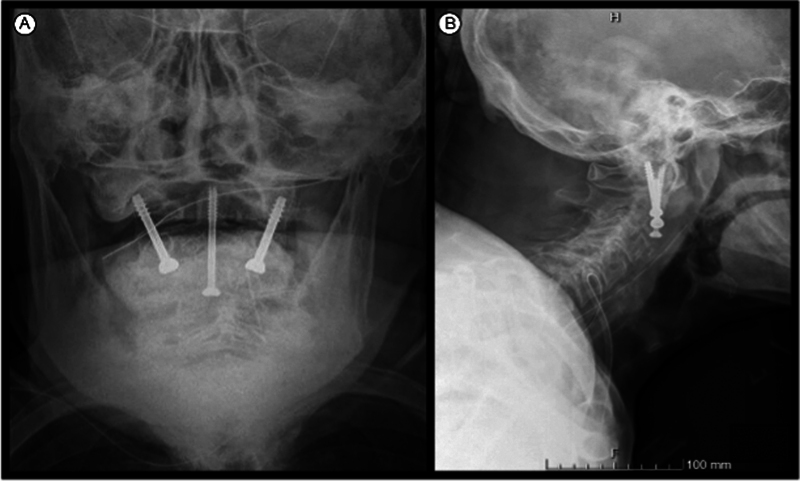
Radiograph of the cervical spine on AP (
